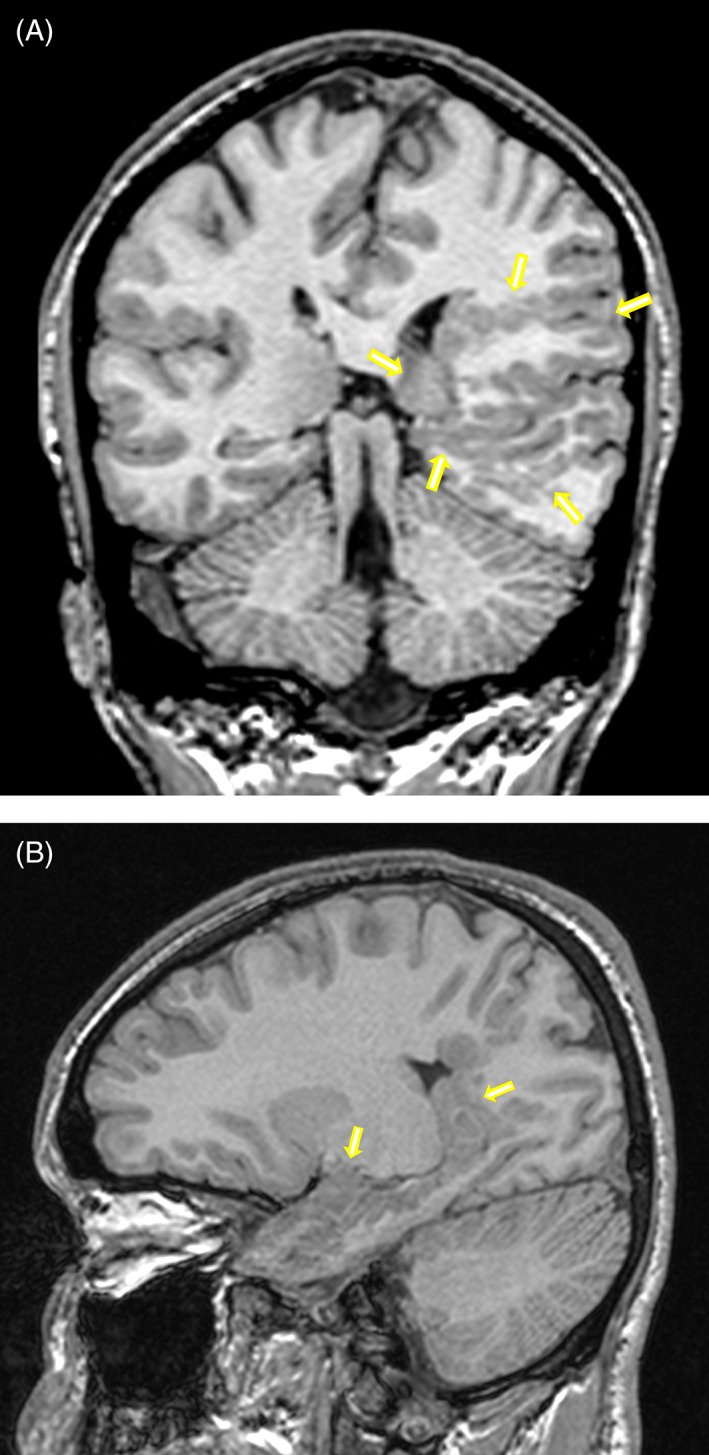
Cerebral magnetic resonance imaging at age 17 years in the carnitine palmitoyltransferase 2 (CPT2)‐deficient patient. Coronal,A, and sagittal, B, 1 mm reformat from a 3D T1 volume acquisition. A, Extensive polymicrogyria, closed lip schizencephaly, and heteropia. B, Extensive heterotopia and abnormal hippocampal formation. The yellow arrows indicate the edge of the malformation
